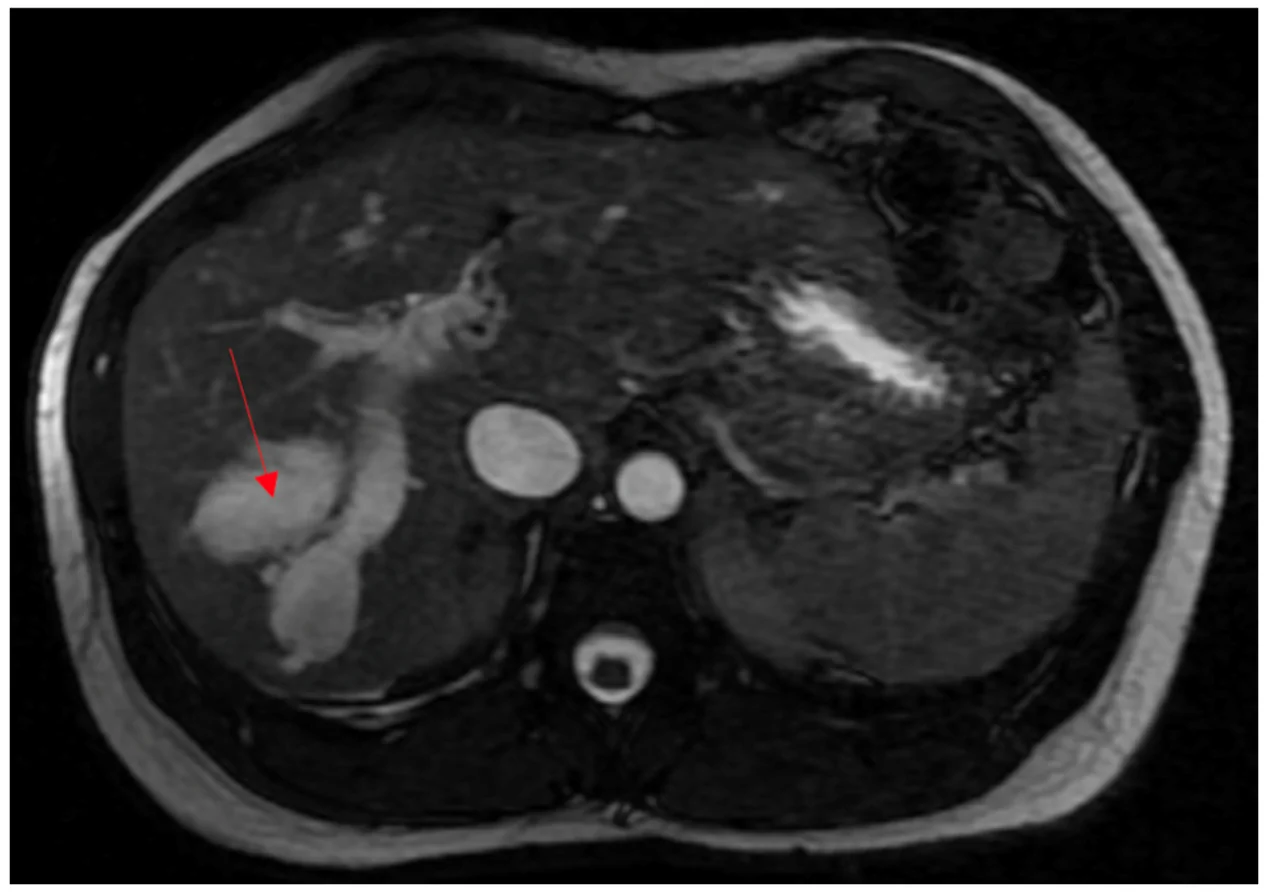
Axial view of MRI showing presence of an intrahepatic portosystemic venous shunt (arrow).
